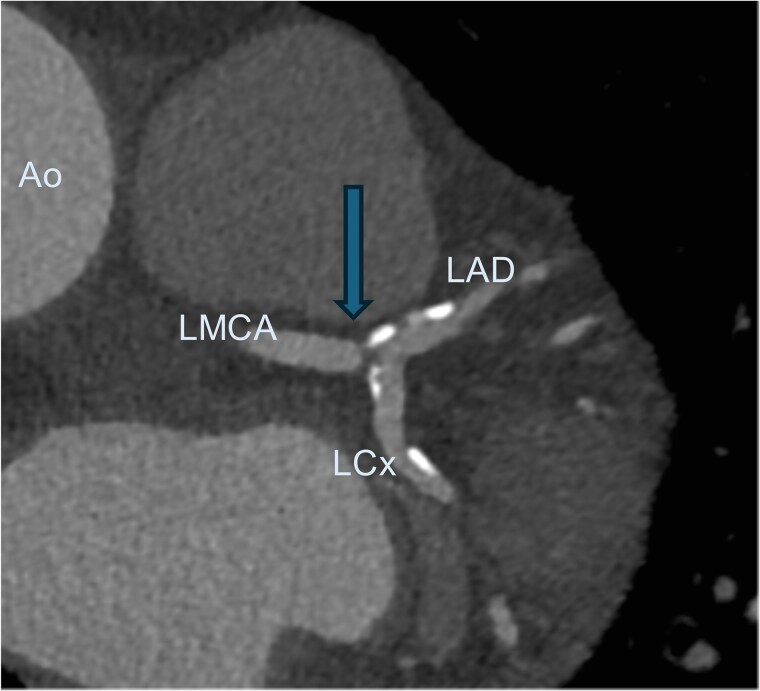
Coronary computed tomography angiography showing left main coronary artery bifurcation stenosis (arrow). Ao, ascending aorta; LAD, left anterior descending artery; LCx, left circumflex artery; LMCA, left main coronary artery.
